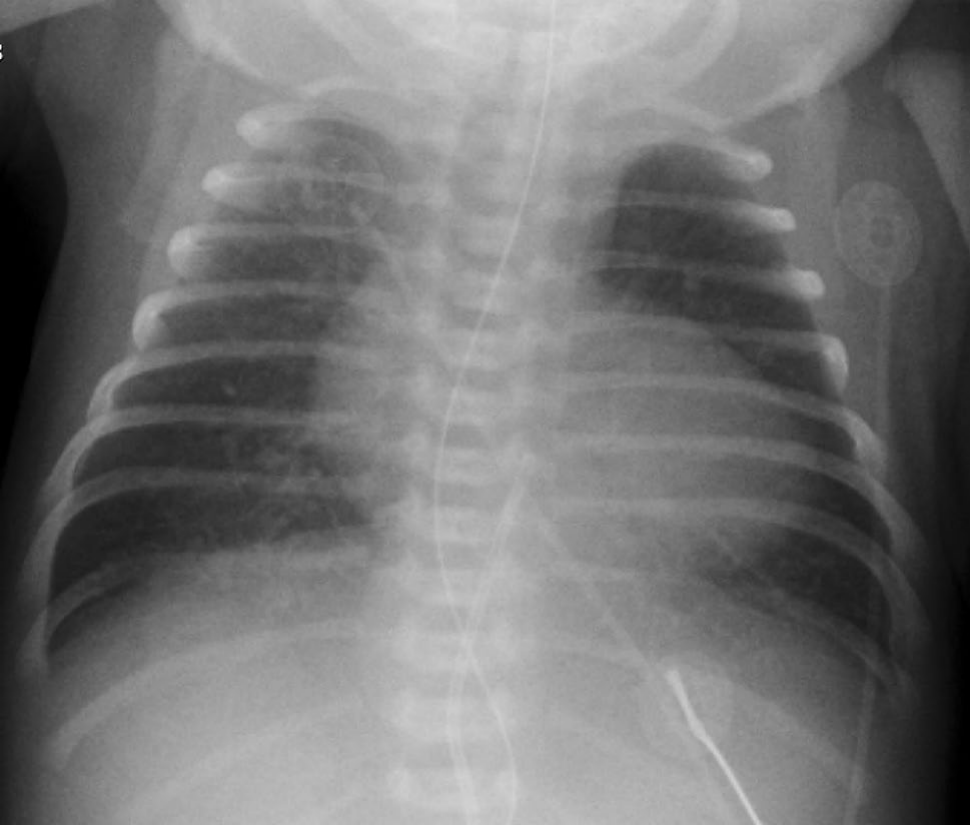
Chest X-ray, suggesting the UVC was positioned rather deep and to the left
